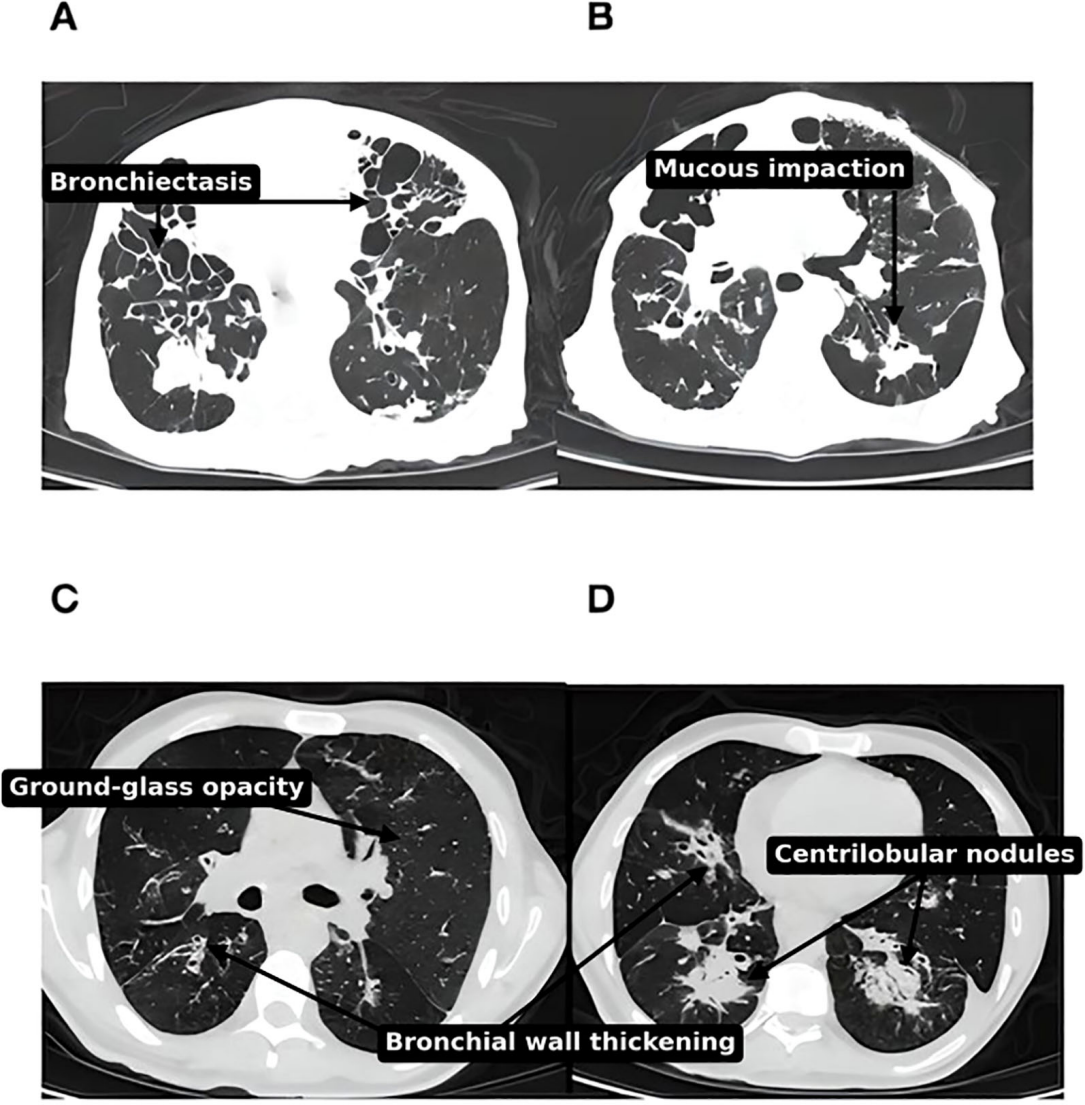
(A, B) Thoracic CT scan, parenchymal window, bilateral diffuse nodules and diffuse cystic bronchiectasis in a 78 years old Moroccan patient with CVID (no gene was identified). (C, D) Thoracic CT scan, parenchymal window, of diffuse nodules, reticulations and ground-glass opacities. Apart from CVID-ILD features, there are also diffuse bronchiectasis with mucoid impaction pointing to surinfection, in 55 years old male Moroccan patient with *TNFRSF13B* mutation.
